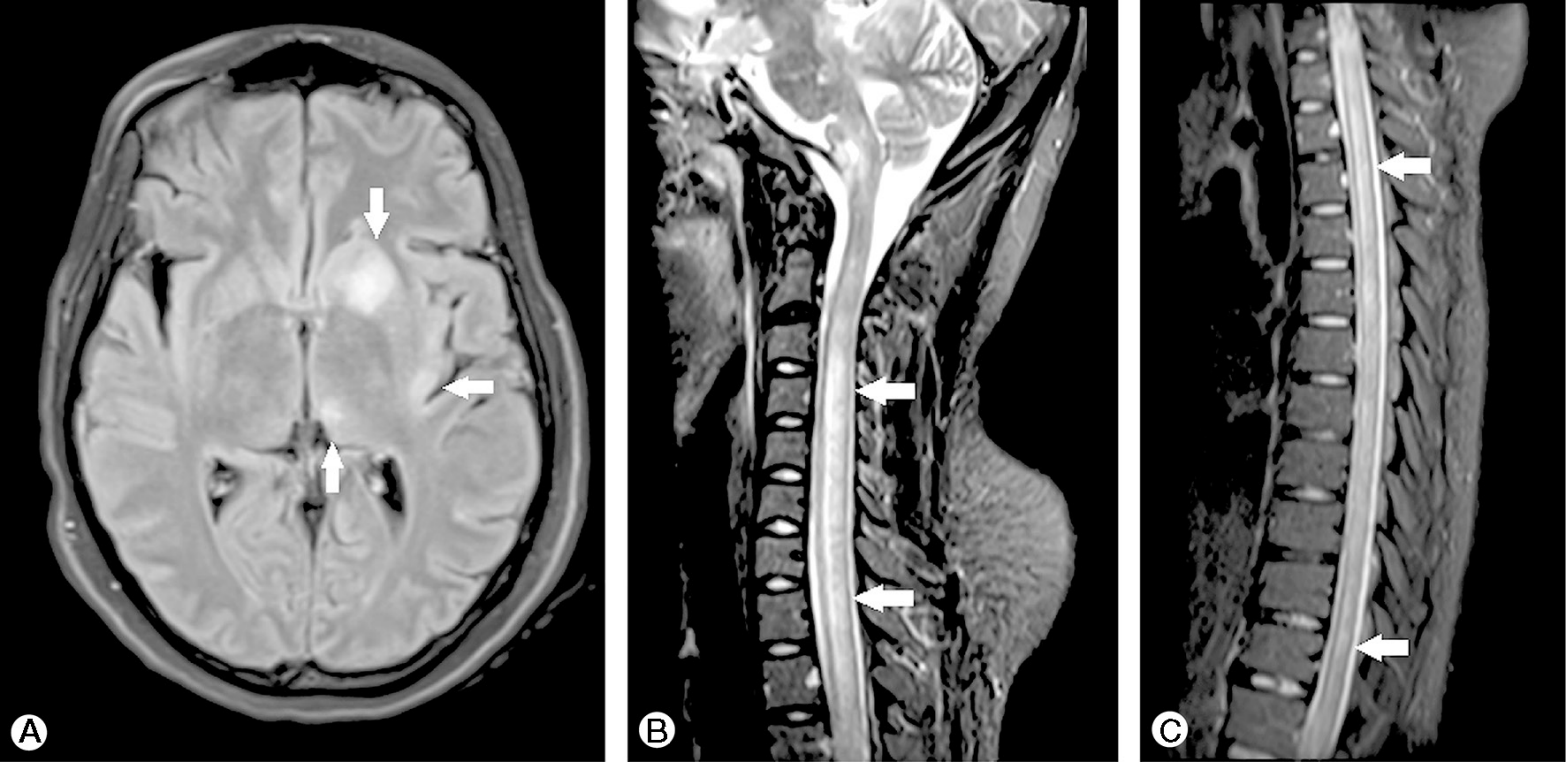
MR scan of brain fluid-attenuated inversion recovery (FLAIR) (A) and short tau inversion recovery (STIR) (B, C) showing asymmetrical hyperintensities affecting the left nucleocapsular region, thalamus and insula (A), and hyperintense longitudinally extensive lesions in the spinal cord. (Copyright © S Karger AG, Basel; Niemeyer *et al*^36^).
